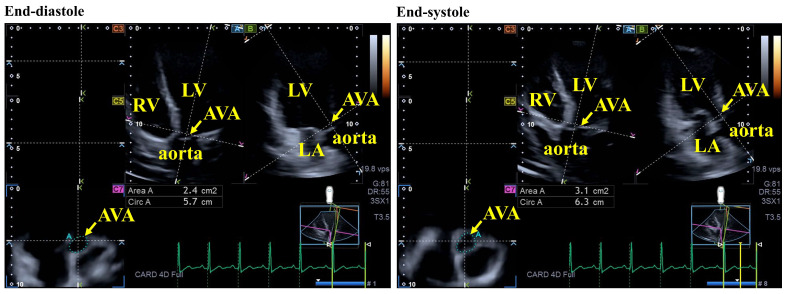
Evaluation of end-diastolic and end-systolic aortic valve annular (AVA) dimensions in apical four-chamber (A) and two-chamber (B) long-axis views by three-dimensional speckle-tracking echocardiography. ‘En-face’ view of the AVA is also presented in C7 cross-sectional view. Spatial AVA displacement is quantified by AVA plane systolic excursion. Abbreviations: AVA = aortic valve annulus, Area A = AVA area, Circ A = AVA perimeter, LA = left atrium, LV = left ventricle, RV = right ventricle.
